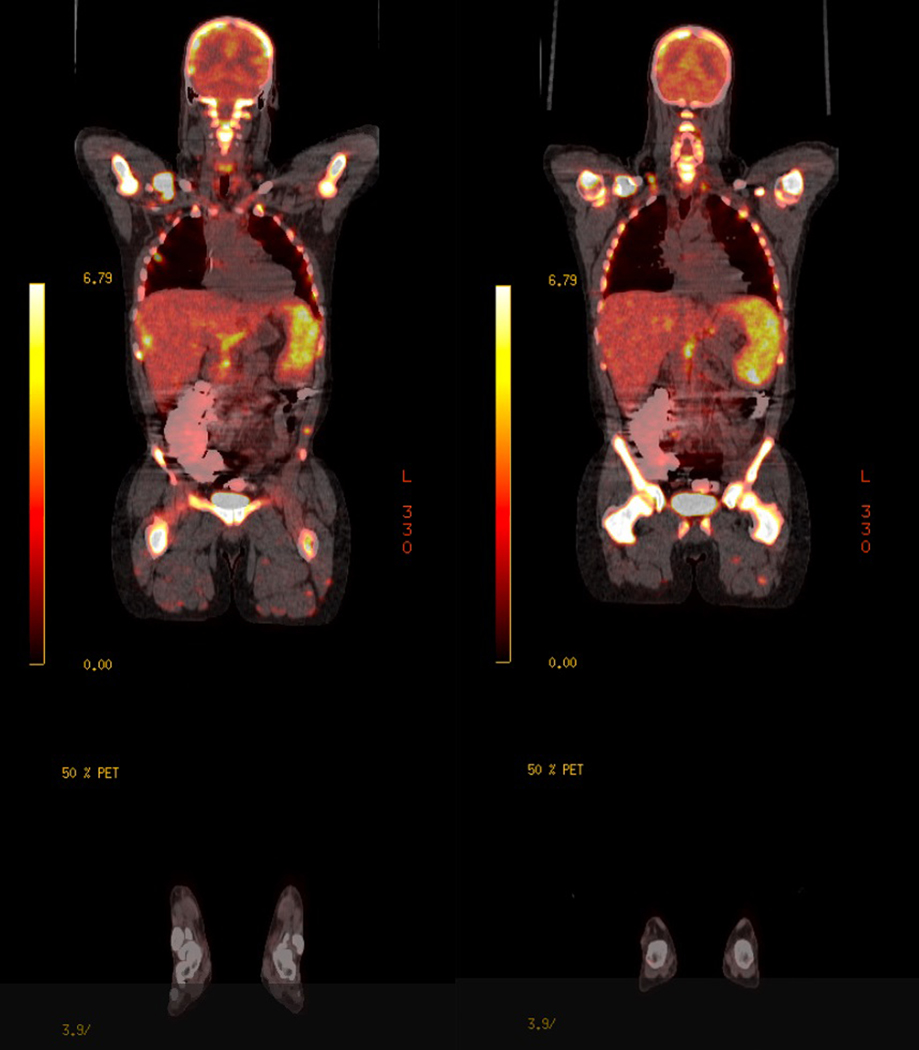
18F-FDG PET/CT in one patient with multiple myeloma 18F-FDG PET/CT scan demonstrates intense hypermetabolic FDG activity in patient with anaplastic multiple myeloma. 18F-FDG, 18F-flurodeoxyglucose, PET, positron emission tomography; CT, computed tomography.
